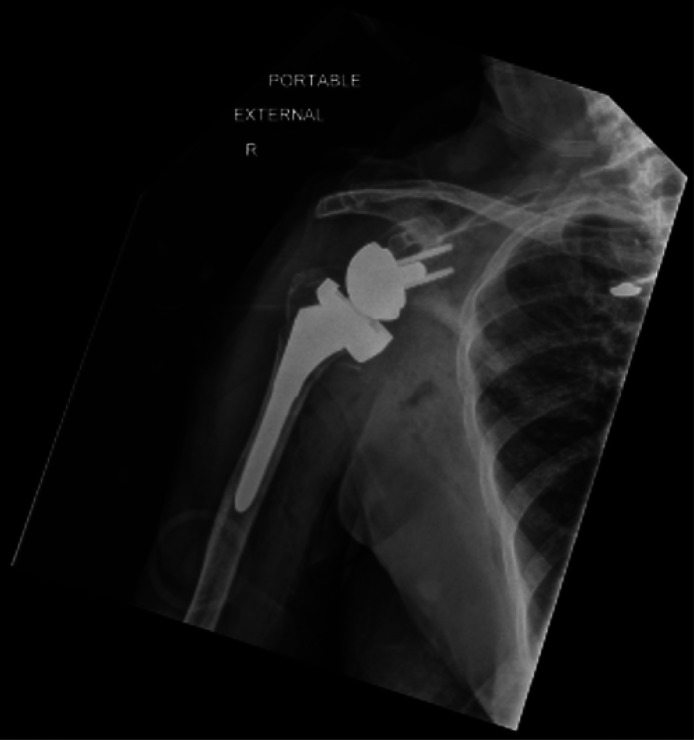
Radiograph of right shoulder 13 days post-dislocation following outpatient, right reverse total shoulder arthroplasty.
